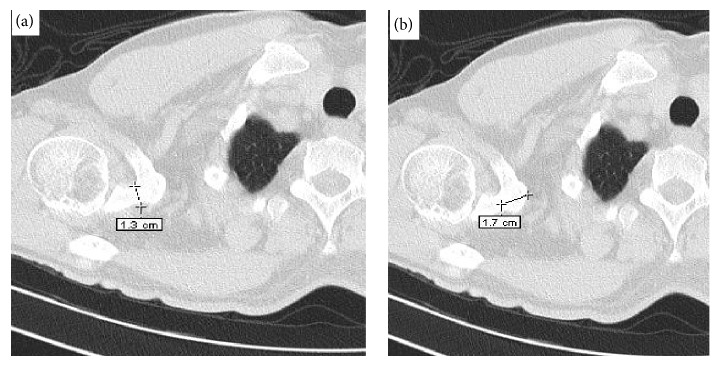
Axial CT scan images showing the measurement of the base of the coracoid process.
